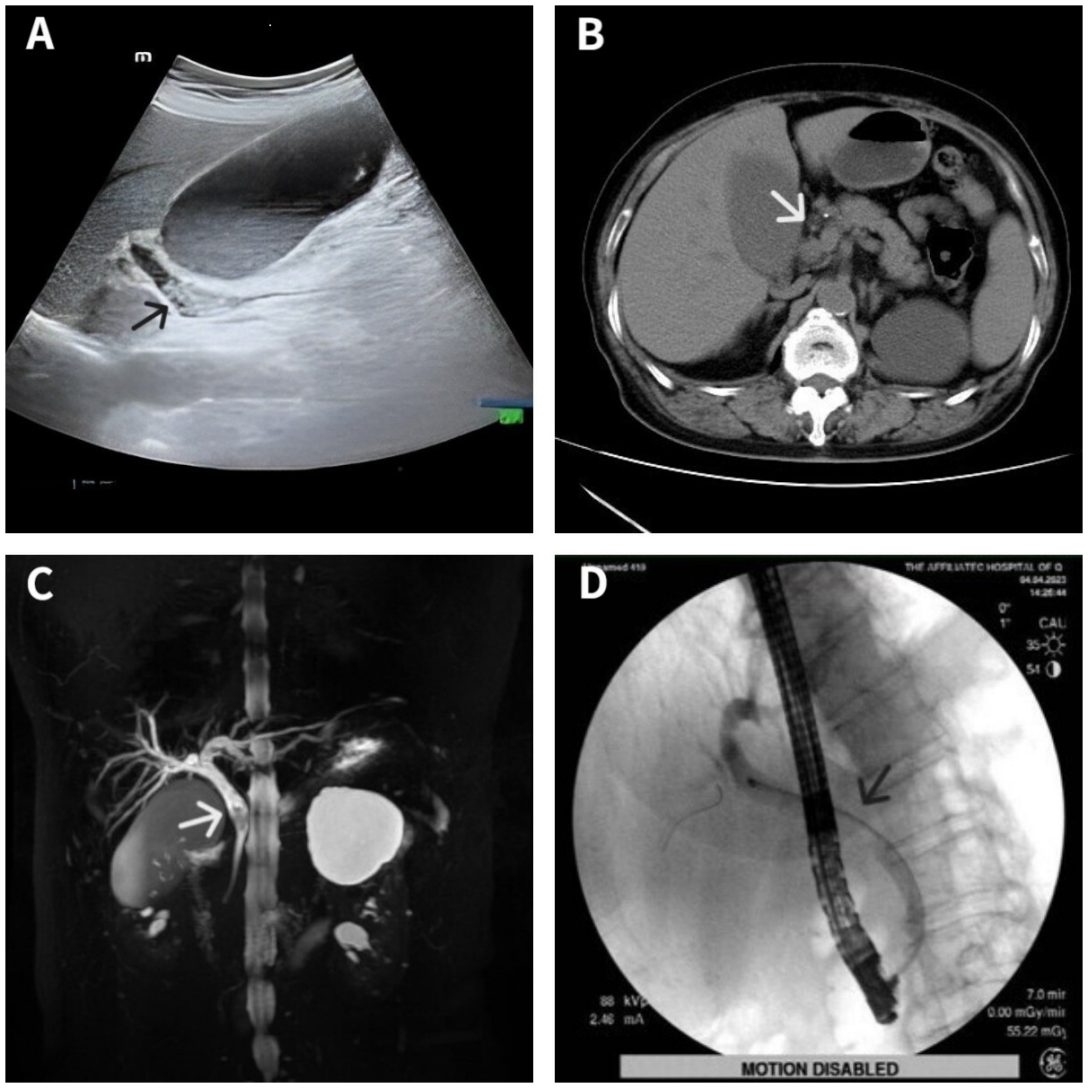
Imaging data of the patient. Ultrasonography showed several hyperechoic masses within the dilated bile duct (A). Computed tomography showed some slightly high-density masses in the bile duct (B). Magnetic resonance cholangiopancreatography showed some hypointense masses within the dilated bile duct (C). Endoscopic retrograde cholangiopancreatography showed a filling defect at the middle bile duct after several stones were removed (D). Lesion locations are marked with arrows.
